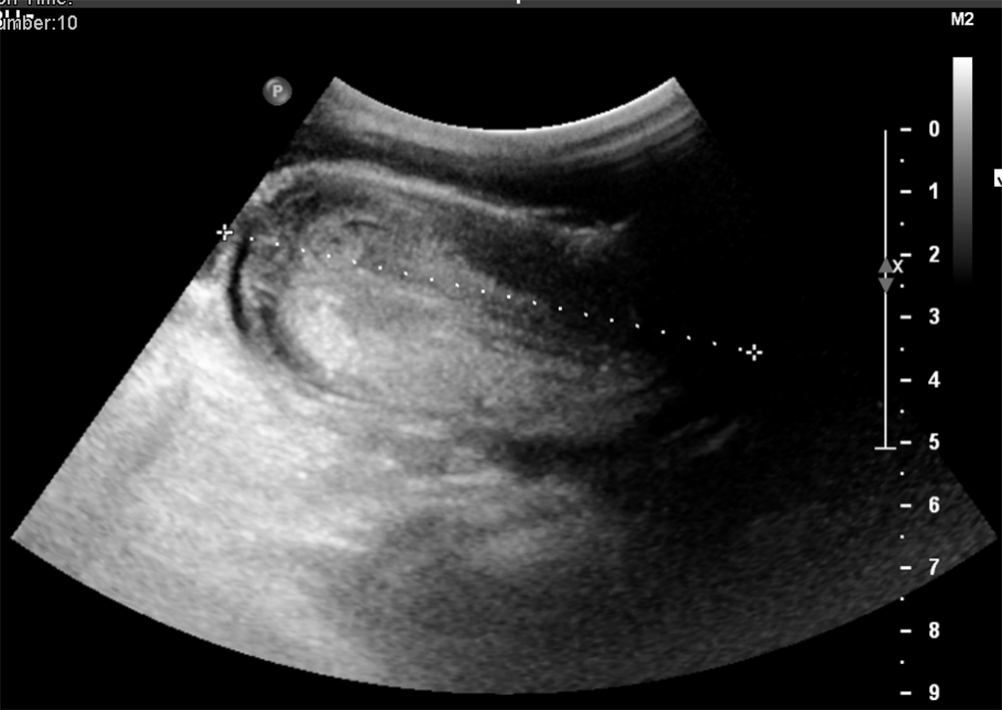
Longitudinal view of intussuscepted bowel on ultrasound measuring 8.2 cm.
